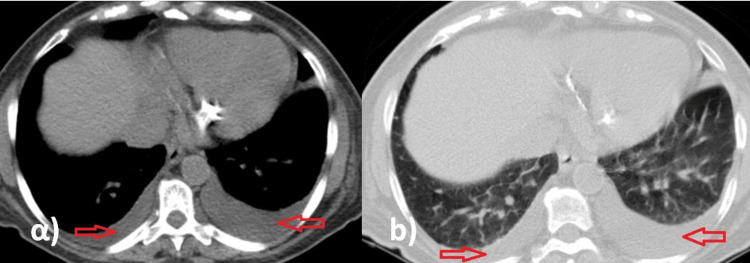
Chest computed tomography scan images. (a) Mediastinal window and (b) lung window reveal bilateral pleural effusion (red arrows).
